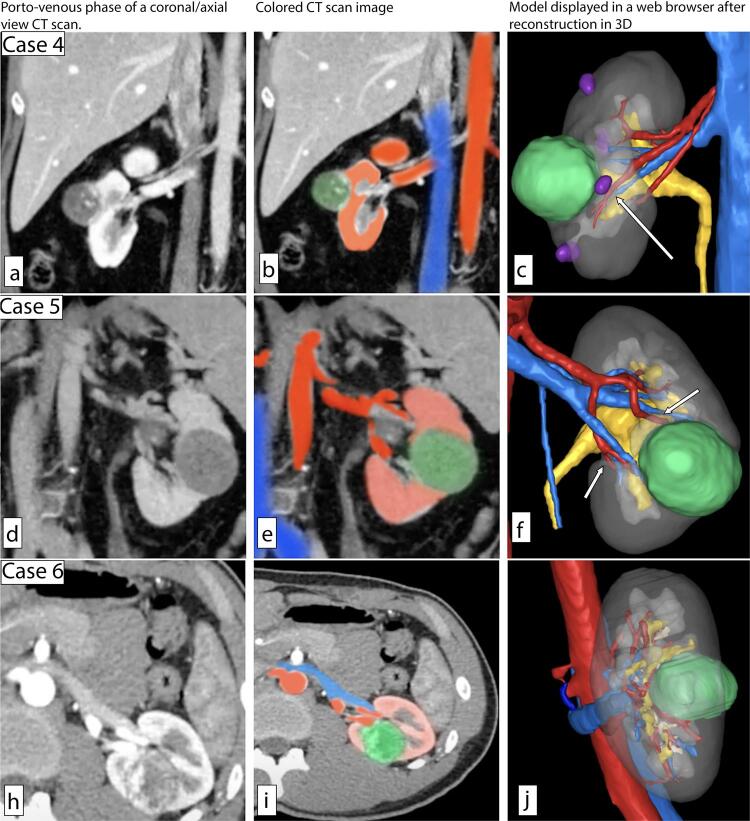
Representative CT scans and 3D reconstructions of the first three surveyed cases. Left, portovenous phase of a coronal/axial view CT scan. Middle, Colored CT scan image, red (artery/renal cortex), blue (vein), green (mass). Right, Model displayed in a web browser after reconstruction in 3D, red (artery), blue (vein), green (mass), purple (cyst), yellow (collecting system/ureter).
